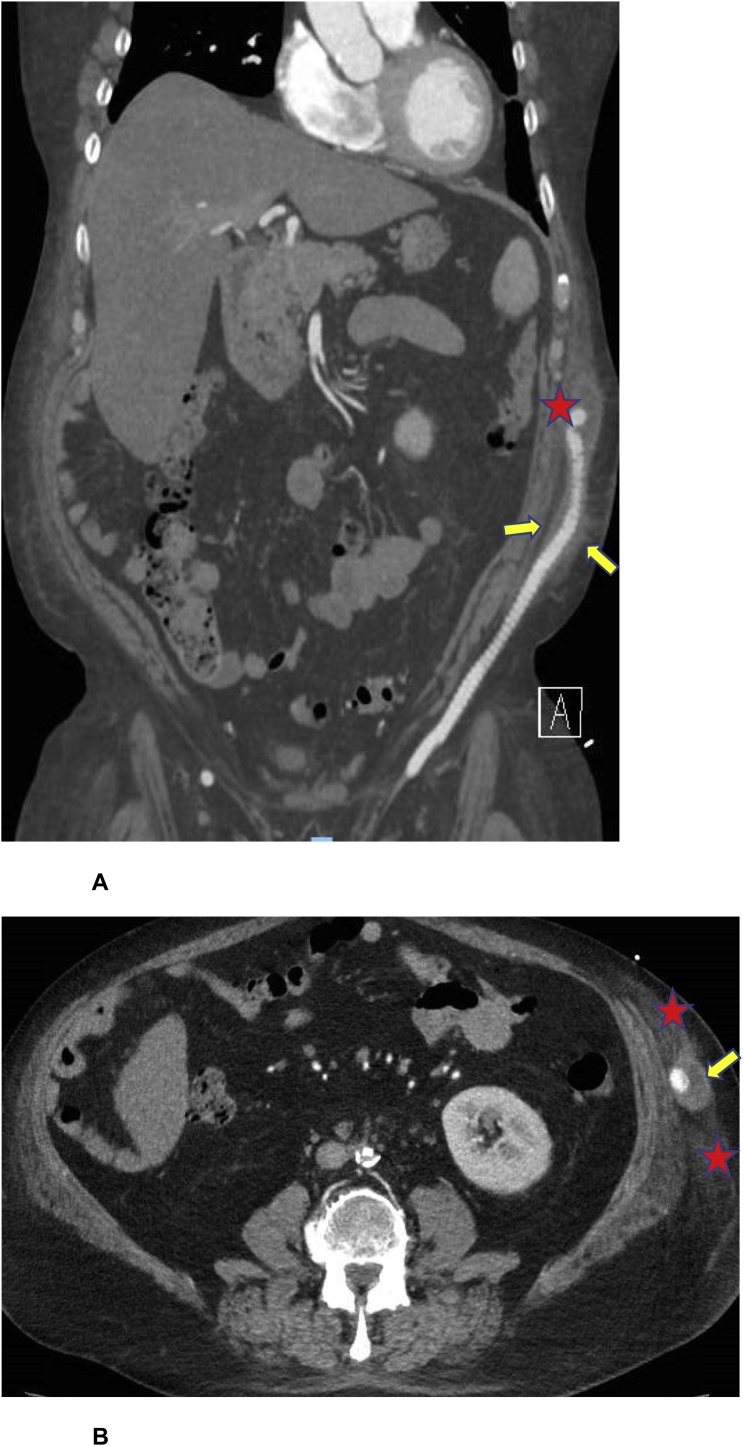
A. Computed tomography angiography (*coronal view*) showing a left axillary to femoral artery bypass with fat stranding (*arrows*) and a small surrounding rim enhancing fluid collection (*star*) extending. B. Computed tomography angiography (*transversal view*) showing a part of a left axillary to femoral artery bypass with fat stranding (*stars*) and a small surrounding rim enhancing fluid collection (*arrow*).
